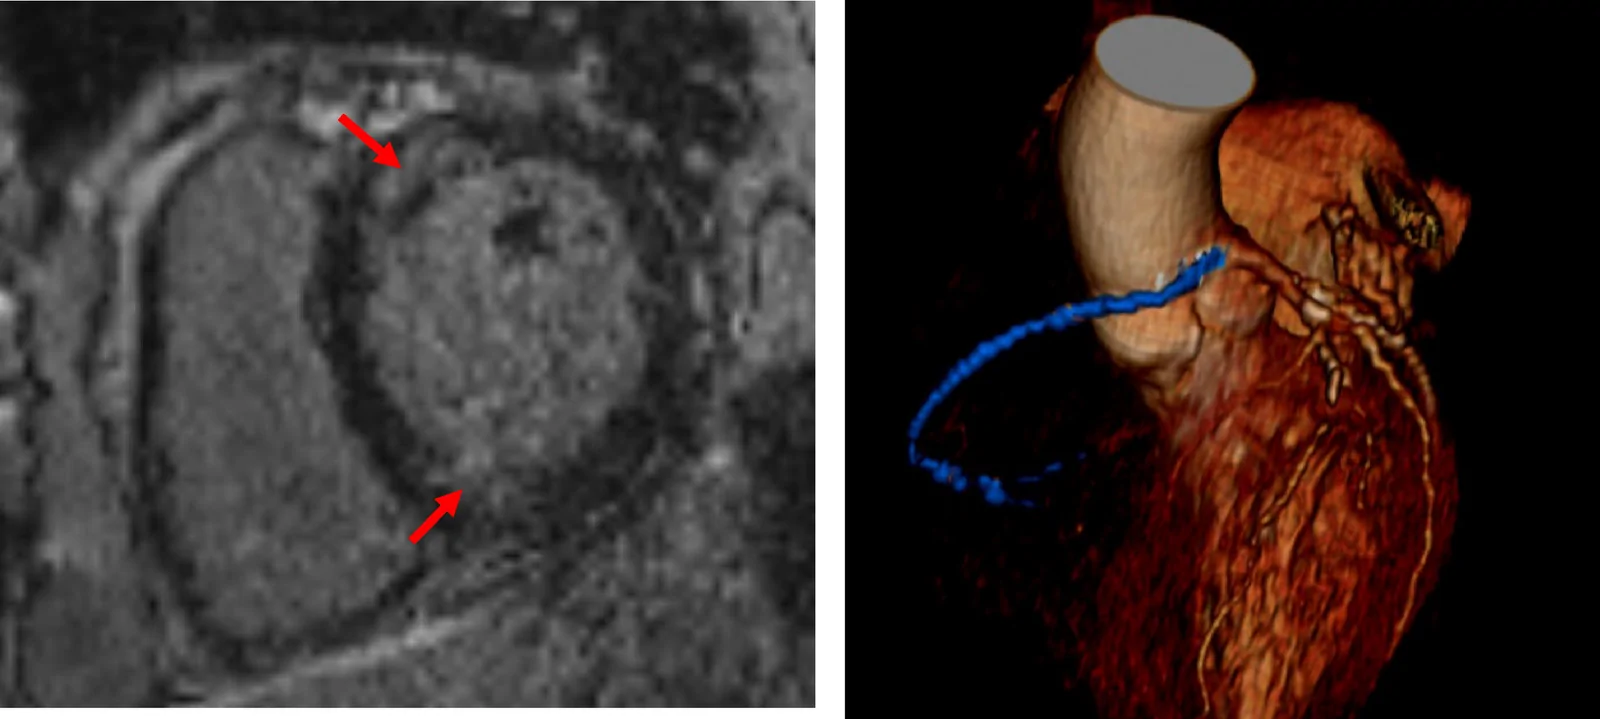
CMR and 3D reconstruction of CT images showing previous infarctions in RCA and LAD territories and an aberant course of the right coronary artery. Left: CMR late gadolinium enhancement image showing a short axis view of the left and right ventricle at the basal level with subendocardial LGE basal inferoseptal, anteroseptal, and anterior wall segments (red arrows) consistent with prior infarctions in the RCA and LAD territories. Right: 3D reconstruction of cCTA images of the right coronary artery (RCA, in blue) demonstrating a slit-like origin with a malignant interarterial course between the aortic root and the proximal pulmonary trunk (images made in TeraRecon Intuition Foster City, USA). 3D; three dimensional, CMR: cardiac magnetic resonance, cCTA: coronary computed tomography angiography LGE: late gadolinium enhancement. (For interpretation of the references to colour in this figure legend, the reader is referred to the web version of this article.)
